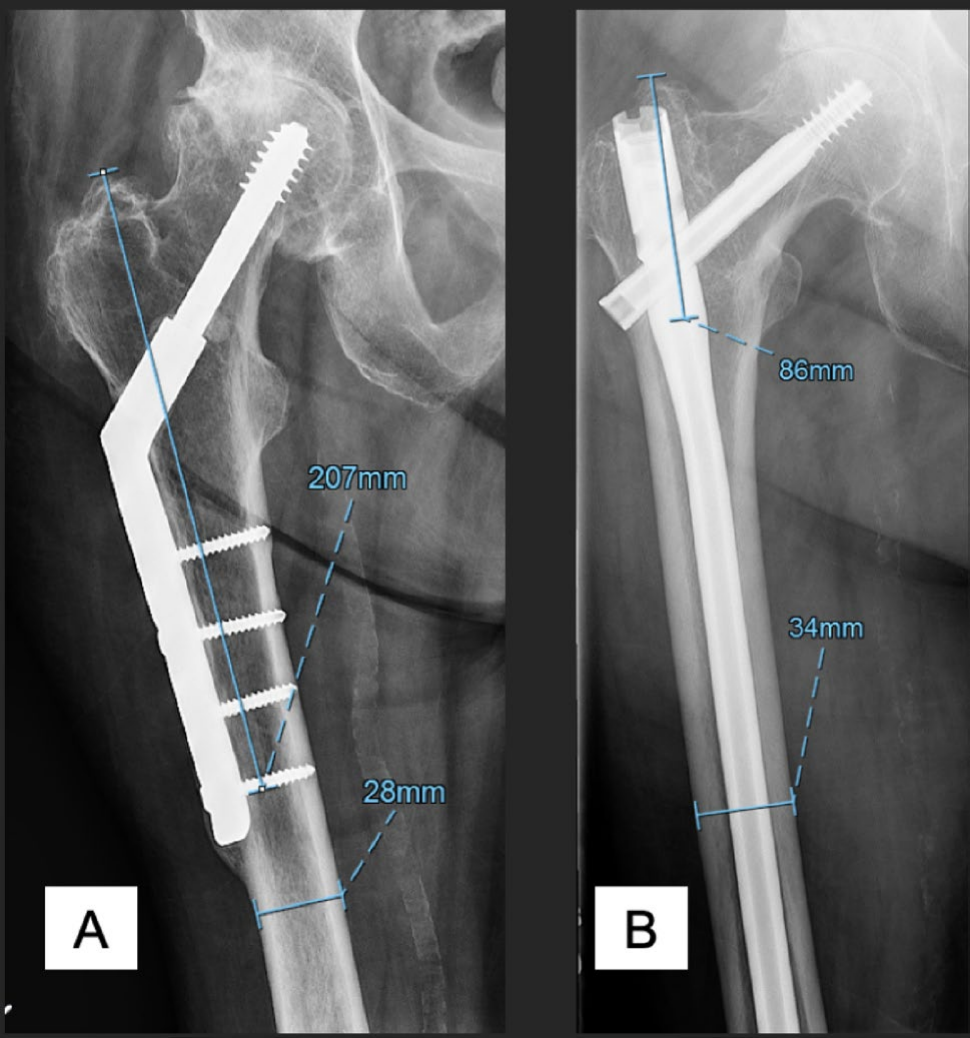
Representative radiographs showing measurement of GSD (distance from the proximal aspect of the greater trochanter to the most distal screw proximal to the femoral isthmus divided by the diaphyseal width) in patients with sliding hip screw and long cephalomedullary nail constructs. GS represents distance from greater trochanter to most distal screw proximal to the femoral isthmus (vertical measurement). Horizontal measurement represents diaphyseal width A. Measurement of GSD in a patient with sliding hip screw fixation B. Measurement of GSD in a patient with long cephalomedullary nail fixation
